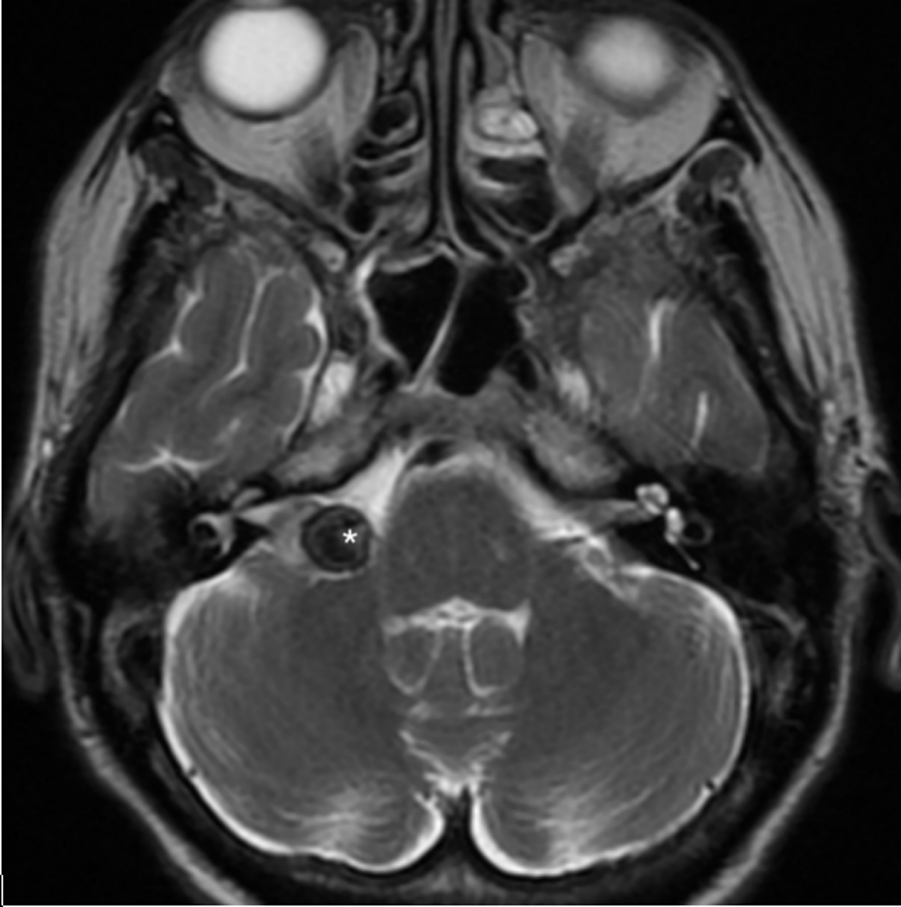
Axial cut of T2-weighted MRI demonstrating hypointense focus within right CPA lesion. MRI: magnetic resonance imaging.
